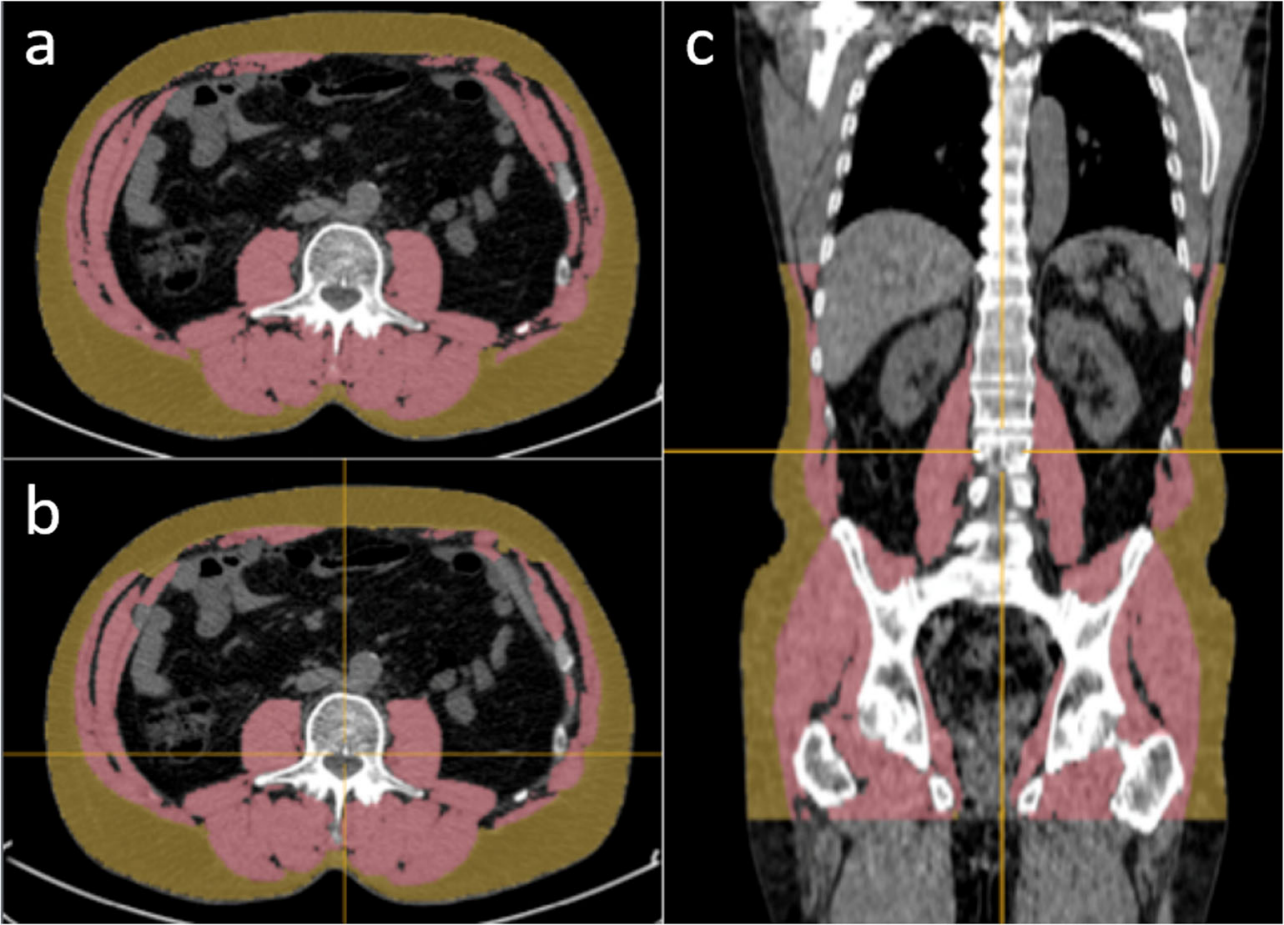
Manual and AI-based segmentations of SAT and muscle. Left: segmentation on a CT slice at L3 level: manual (a) and AI-based (b). Coronal slice showing the AI-based 3D segmentation from T11 to the hip bone (c). Measurements: manual areas, 186 cm^2^ (SAT) and 170 cm^2^ (muscle); AI-based areas, 184 cm^2^ (SAT) and 158 cm^2^ (muscle); AI-based volumes 6,832 cm^3^ (SAT) and 8,253 cm^3^ (muscle). *AI* Artificial intelligence, *SAT* Subcutaneous fat
